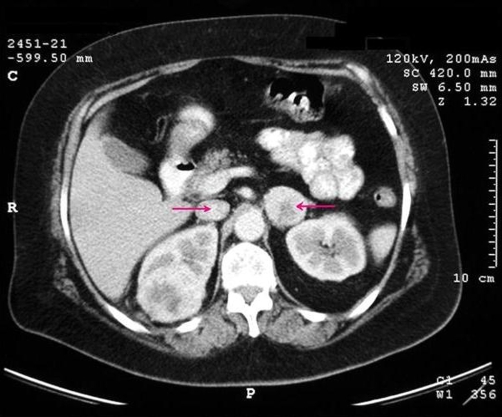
Contrast-enhanced abdominal computed tomography (CT) revealed a 6 cm × 5 cm × 4 cm solid mass at the upper and mid pole of the right kidney that consisted of heterogeneous and enhanced areas and also bilateral involvement of the adrenal glands (arrows).
